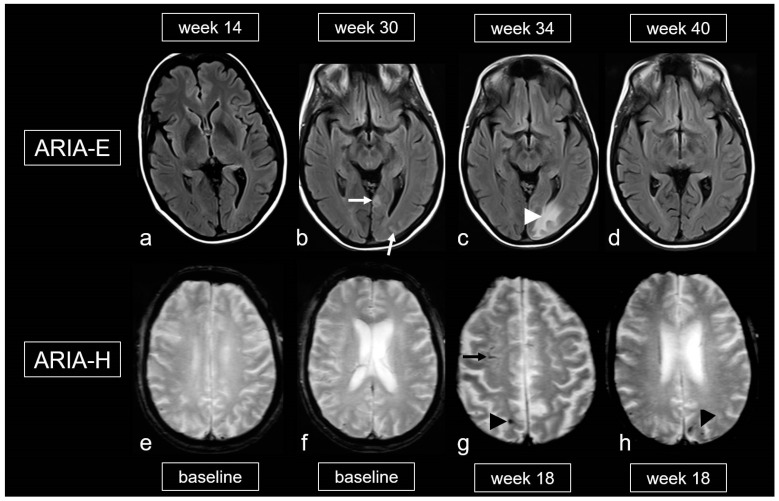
Amyloid-related imaging abnormalities (ARIA). (a–d): Fluid-attenuated inversion recovery (FLAIR) images showing ARIA-E (edema, effusion) in a patient treated with aducanumab, week 14 (a), 30 (b), 34 (c) and 40 (d) after treatment initiation; sulcal effusions ((b), arrow) and edema in the occipital lobe ((c), arrowhead), completely decreased at week 40 (d). (e–h): T2*-weighted images (WI) demonstrating ARIA-H (hemorrhagic) in a 68-year-old woman treated with aducanumab, baseline (e,f) and week 18. (g,h): Cortical superficial siderosis (cSS; arrow) and microbleeds (MB; arrowhead); MRI 1.5T.
